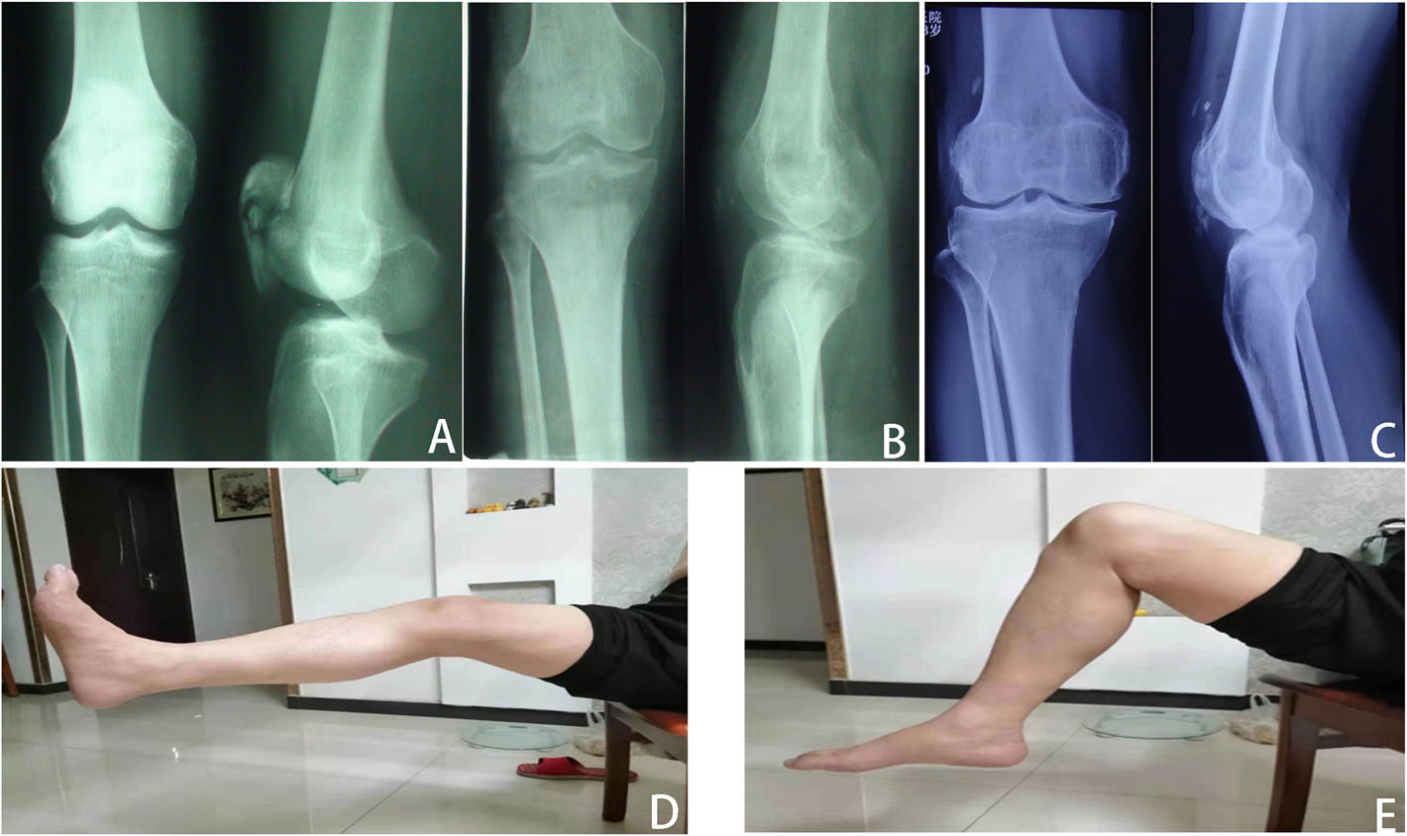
A Male, 52 years old, fall from height, anteroposterior and lateral radiographs of the right knee showed patellar comminuted fractures preoperatively and B postoperative immediately after surgery. C Anteroposterior and lateral radiographs of the left knee postoperatively at the final follow-up. D, E Satisfactory functional outcome was restored normally at the final follow-up
